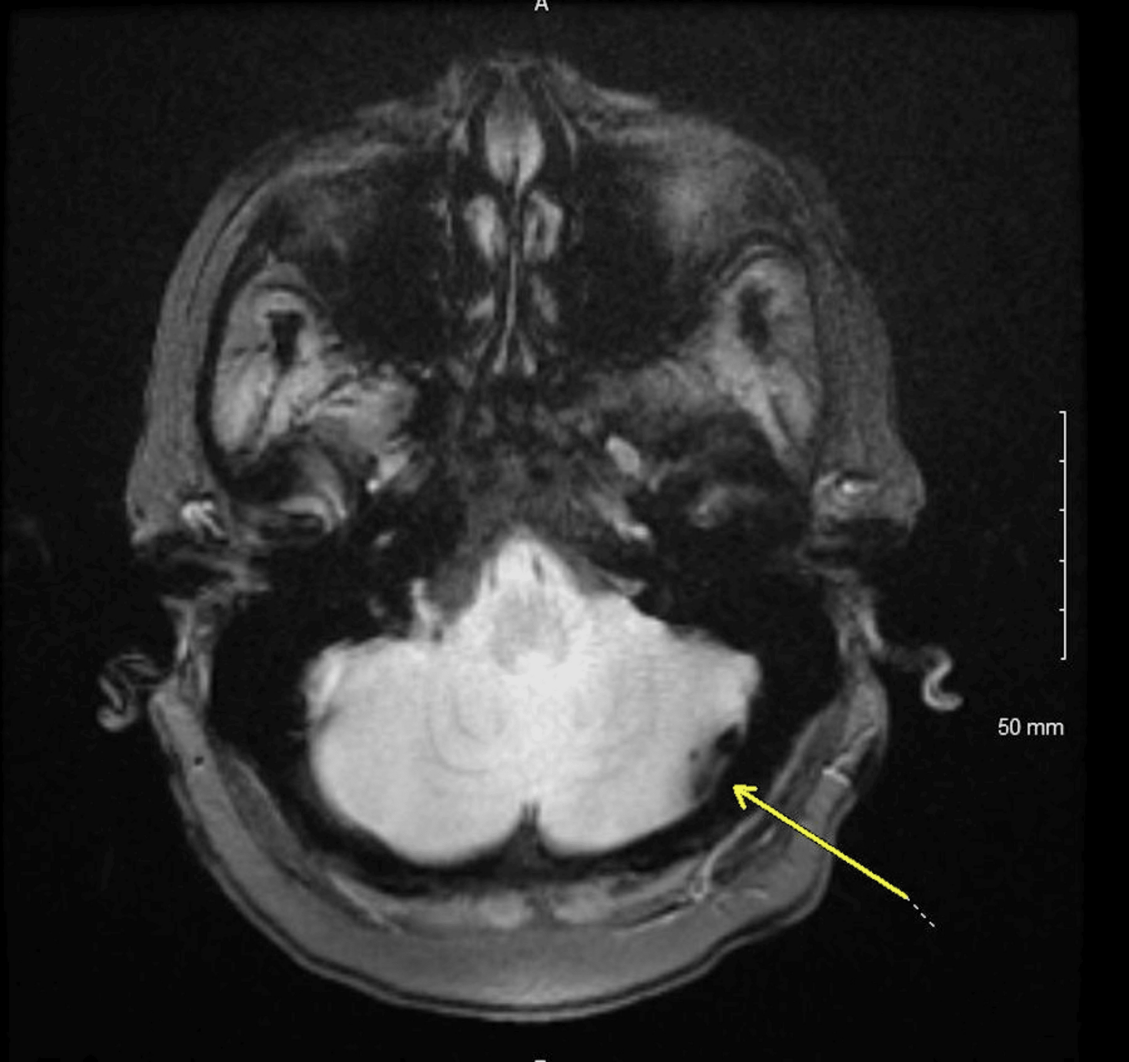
MRI brain without contrast (T2 weighted) with a yellow arrow indicating a small posterior left cerebellar stroke.
